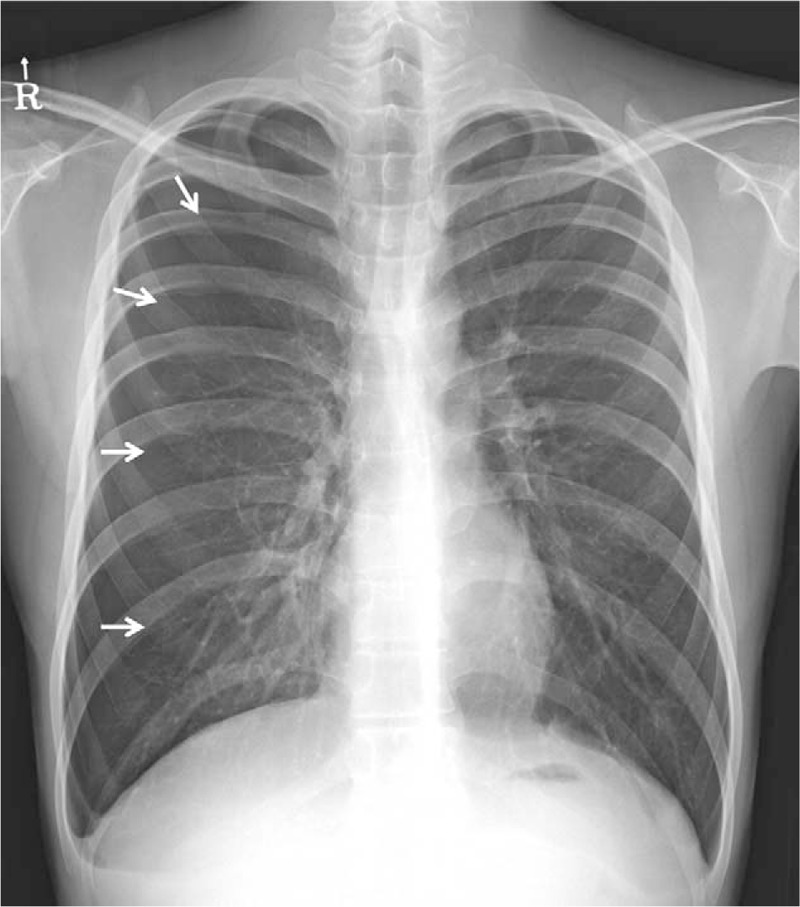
Chest X-ray at presentation. Pneumothorax, which accounts for about 40% of the right hemithorax, was observed. Arrows indicate pleural line.
